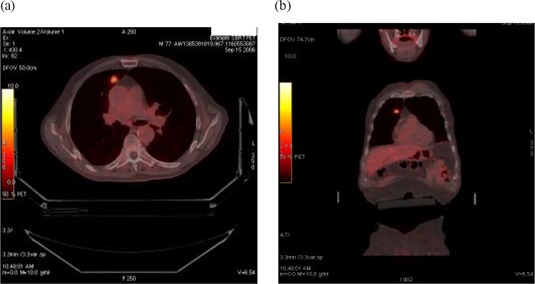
Sample positron‐emission/computed tomography image of a stereotactic body radiation therapy patient.
